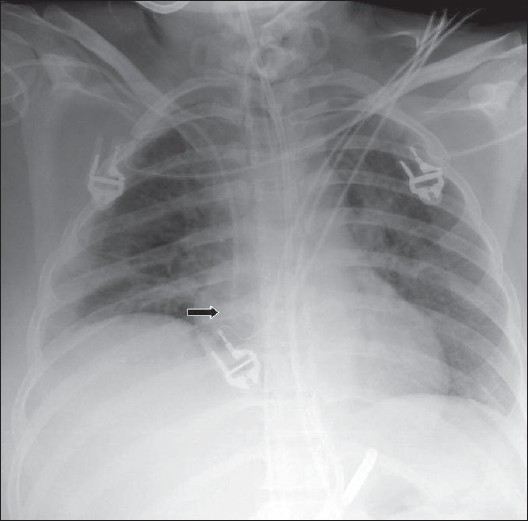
AP chest radiograph showing the tip of an intravenous line within the left atrium (arrow)
